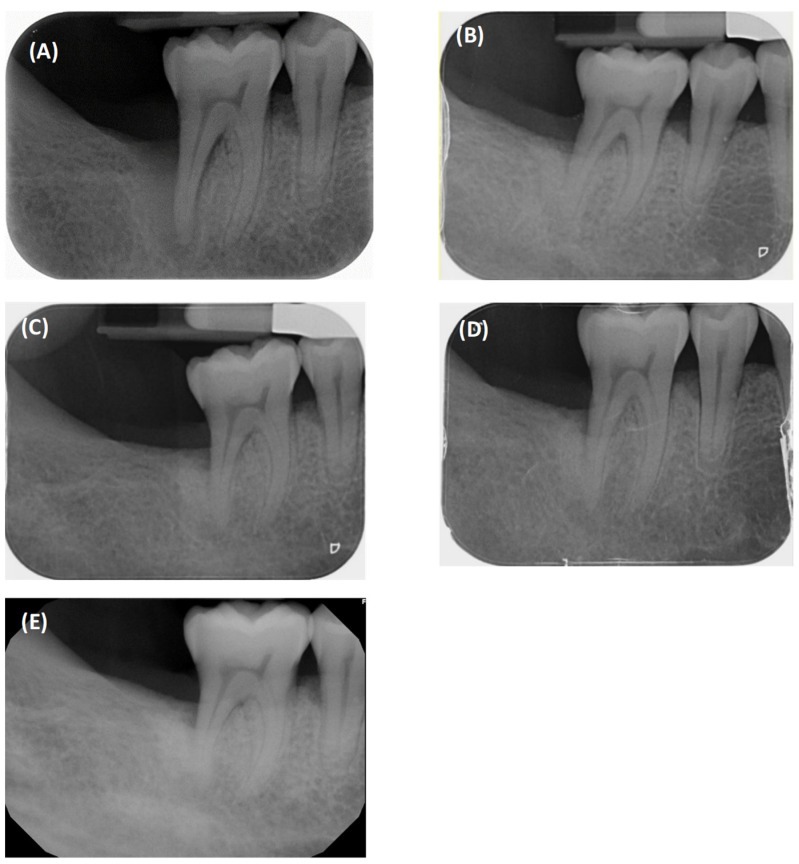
Radiographic follow-up of #46: (A) baseline; (B) 1 year; (C) 2 years; (D) 3.5 years; (E) 5 years.
